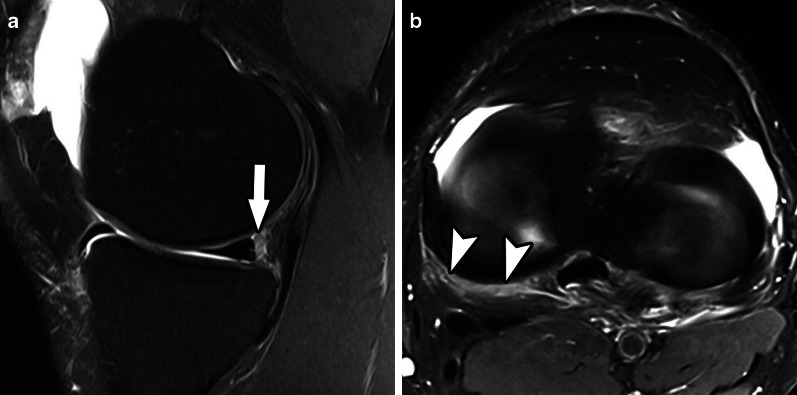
Type 4B meniscal ramp lesion MRI case. Sagittal (a) T2-weighted fat-suppressed MR image shows a type IV ramp lesion, with a complete meniscocapsular disruption of the posterior horn of medial meniscus. Axial (b) image shows a cross-sectional view of the extensive meniscocapsular disruption
